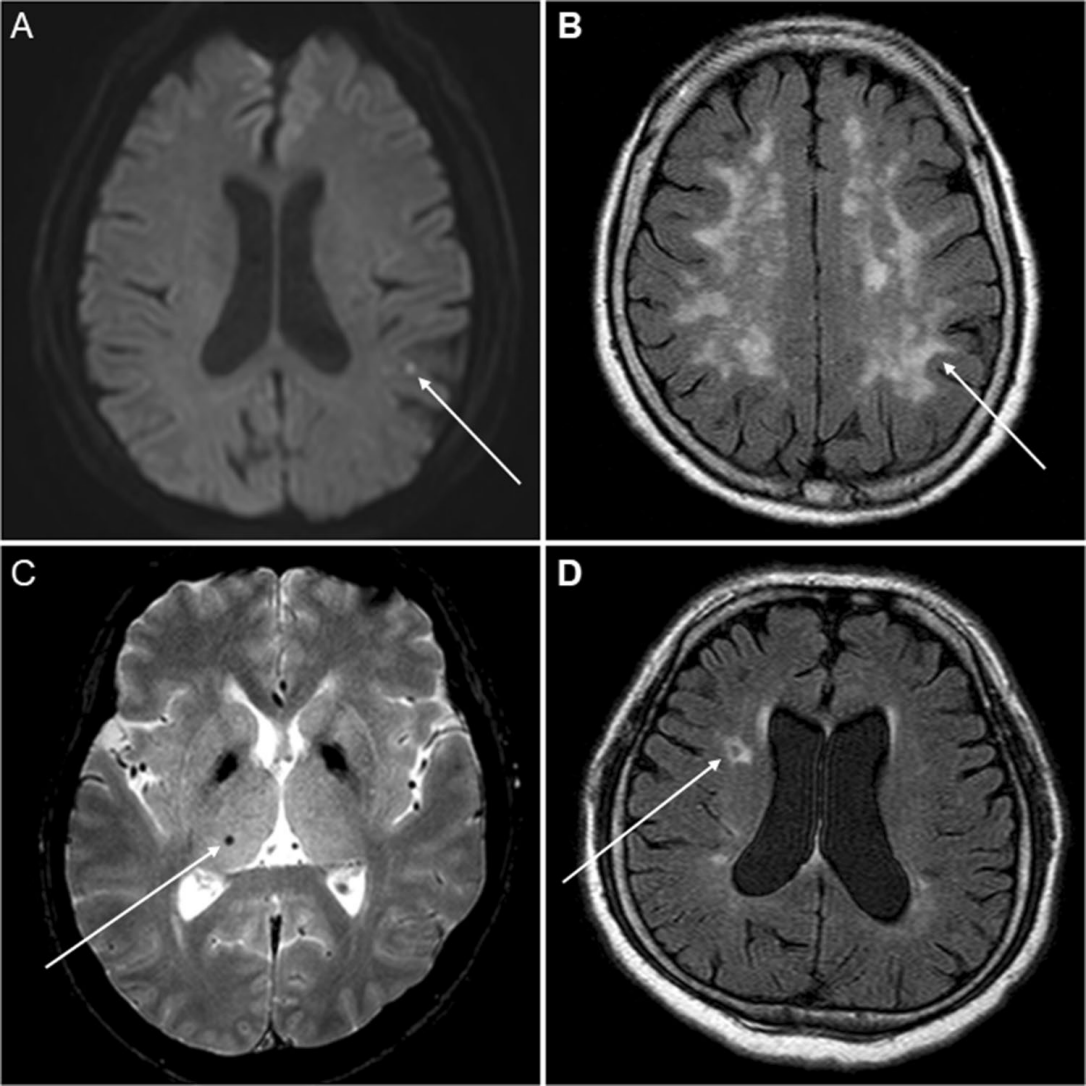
Representative cerebral magnetic resonance imaging of (A). acute infarction, (B). white matter hyperintensity lesion, (C). cerebral microbleeds, and (D). silent lacunar infarction. Arrows indicate the corresponding lesion in each figure.
